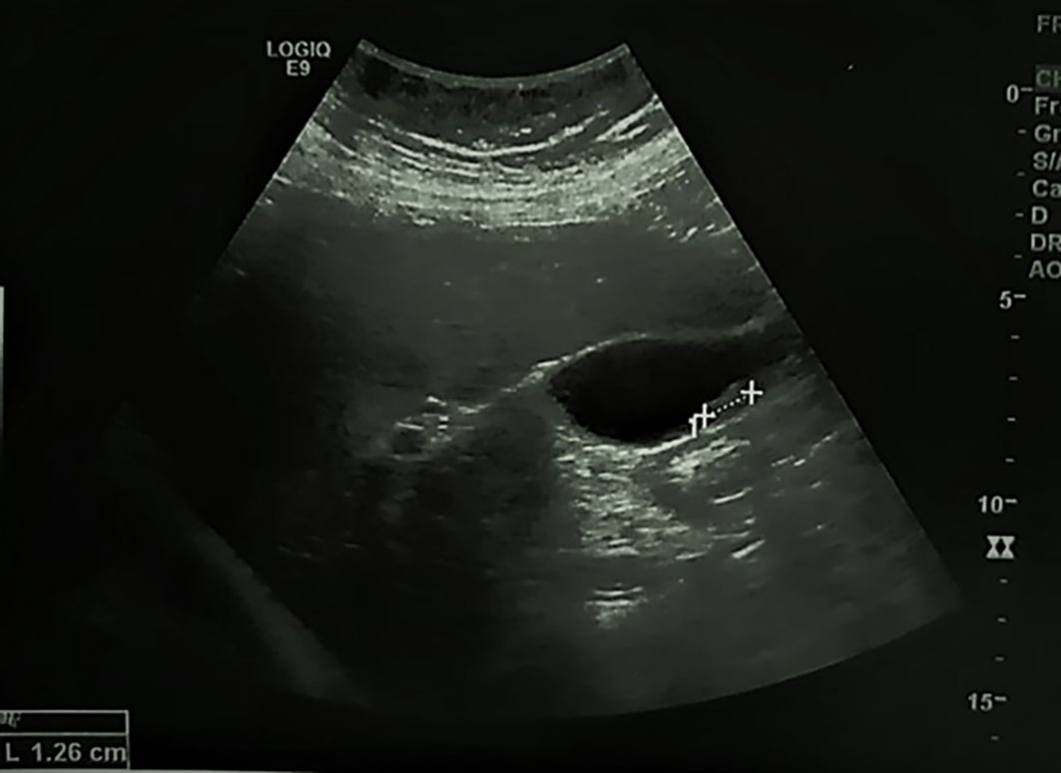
Abdominal ultrasound. The walls of the gallbladder (GB) are thickened, hyperechoic with a mobile gallstone.
